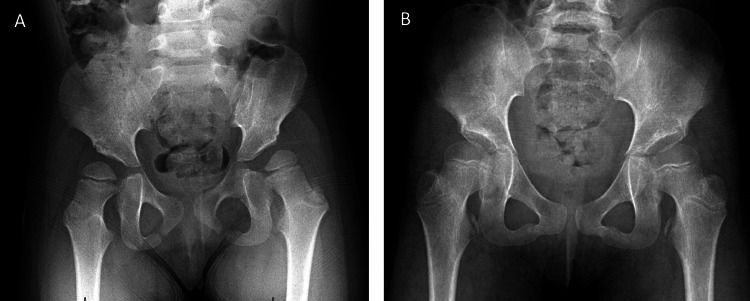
Case 1 X-rays: preoperative (A) and postoperative (B). Gross Motor Function Classification System level 4 initially, later 2. On the preoperative pelvis X-ray, the left hip has a migration percentage (MP) of 60. At four years postoperative, the left hip has an MP of 35.
